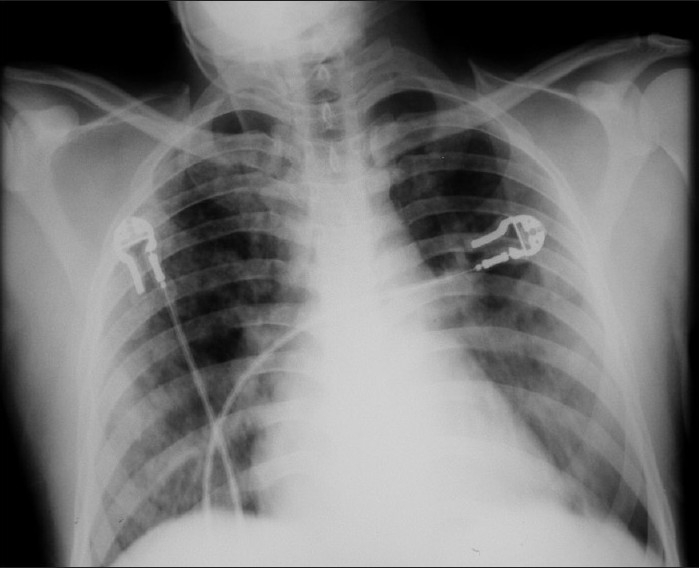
X-rays showing B/L upper and middle zone acinar opacities
